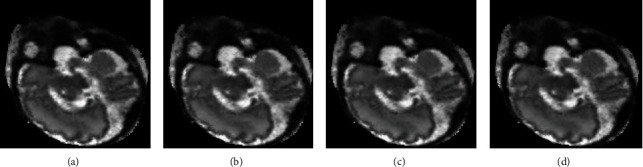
Reconstructed results of the MRI data with different steering smoothing parameters: (a) *h*_s_ = 0.5, (b) *h*_s_ = 1.0, (c) *h*_s_ = 1.5, and (d) *h*_s_ = 2.0.
